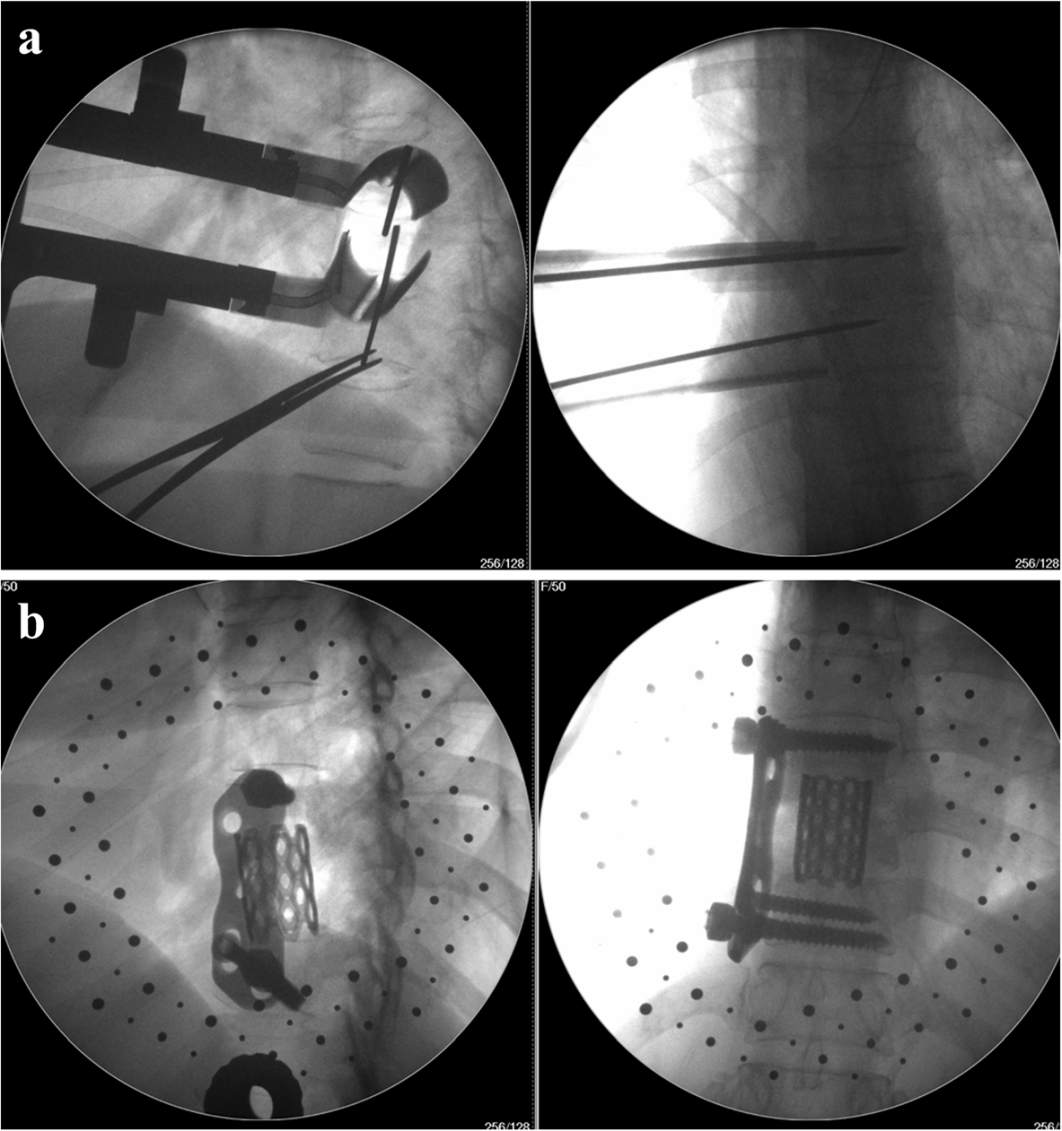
X-ray fluoroscopy of working channel in the operation. a The working channel established in the operation. b Postoperative X-ray after withdrawal the working channel
